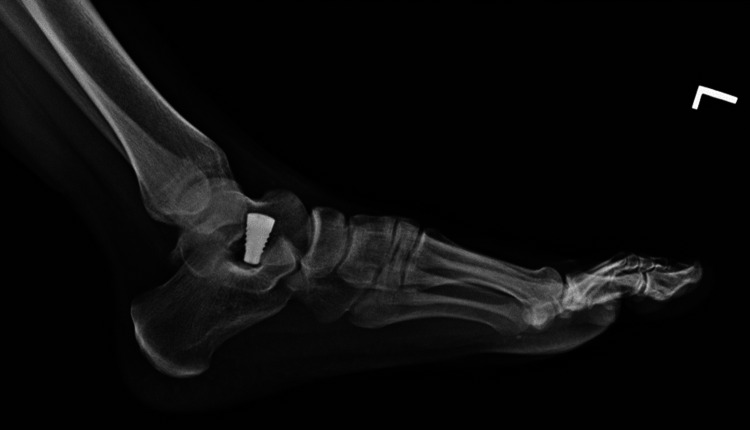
Postoperative lateral left foot X-ray with sinus tarsi implant.
